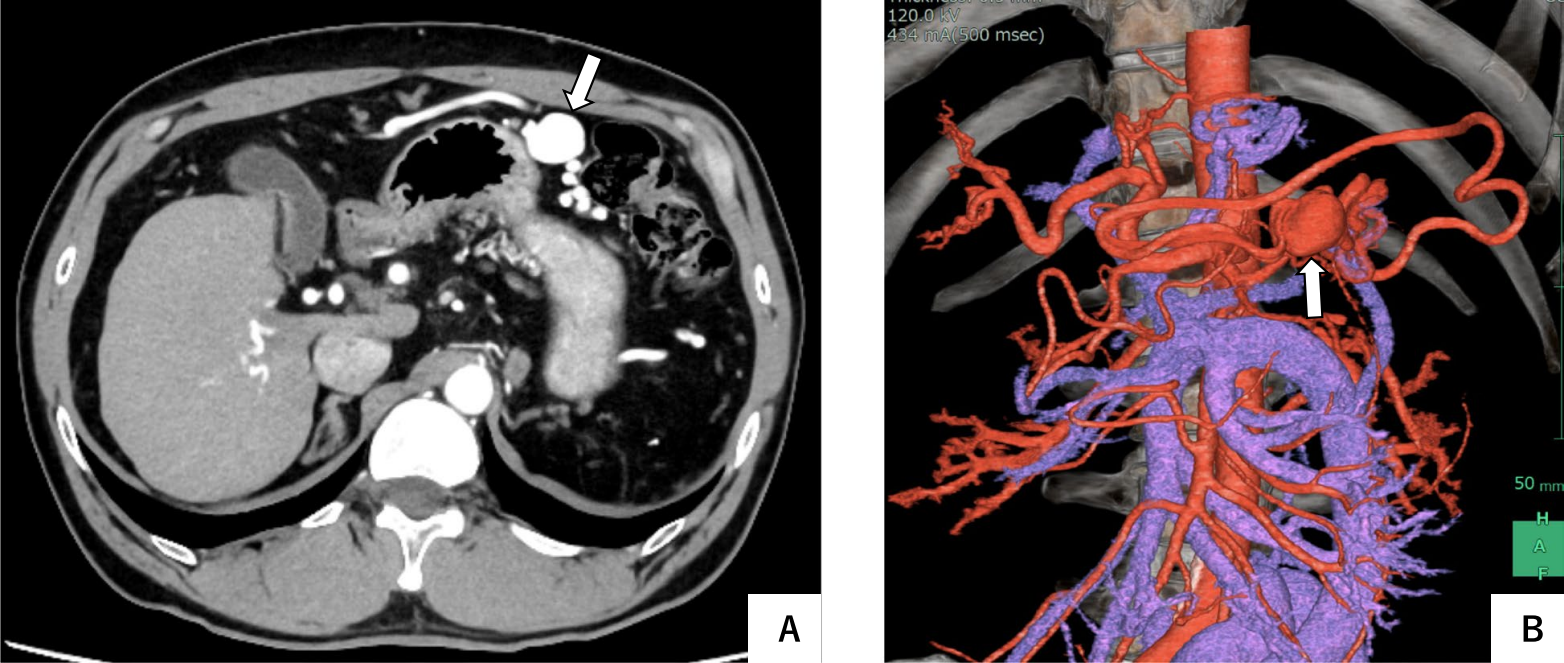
Abdominal contrast-enhanced CT showed an aneurysm-like saccular dilatation in the left upper abdomen (white arrow). A Arterial phase; B VR image
